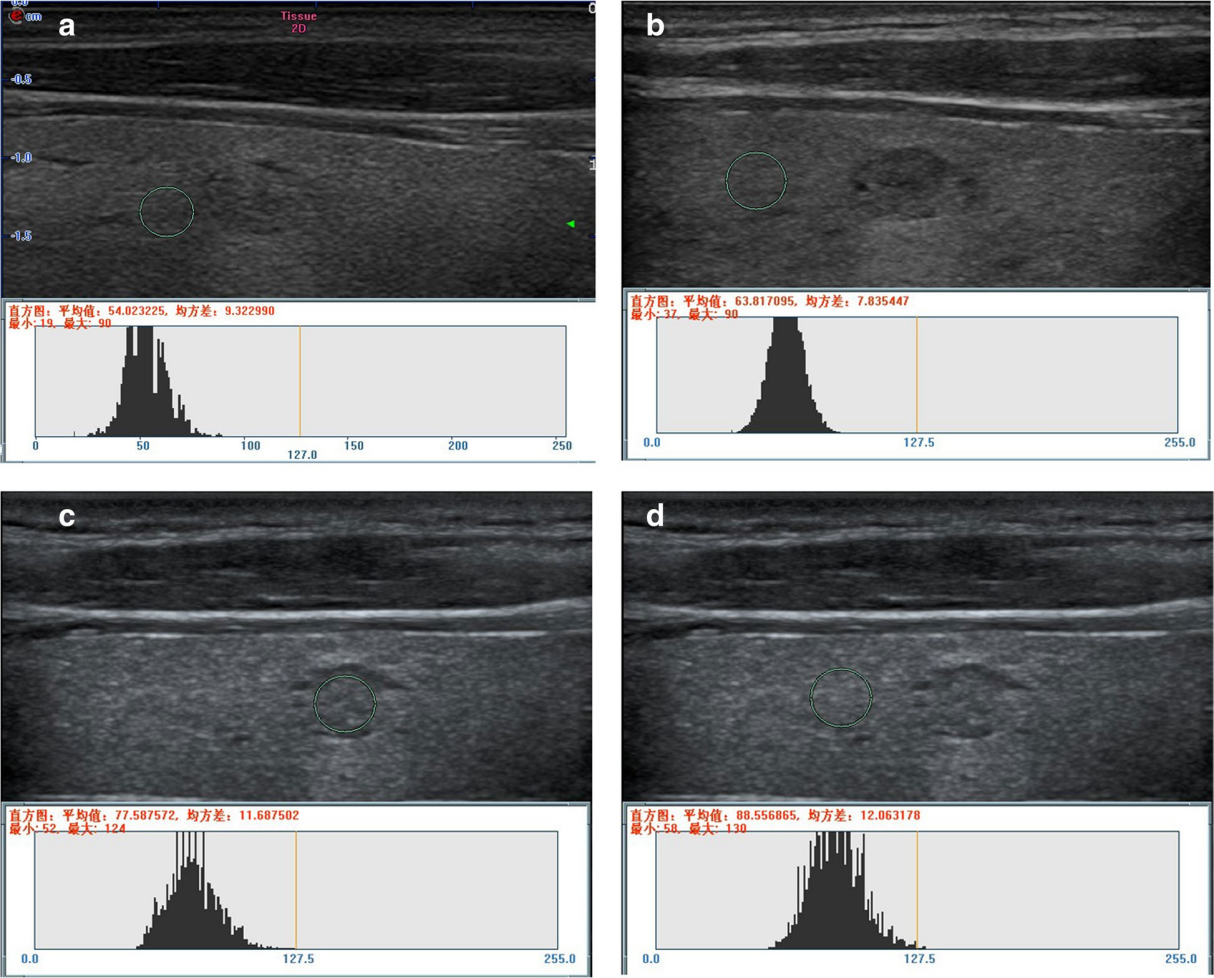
Measurement of the UGSR value for MNG on the left lobe of the thyroid. a and b (ultrasonic apparatus: Mindray Resona7): In the outpatient examination group, a longitudinal scan revealed grayscales of 54.667273 and 63.817095 for MNG and thyroid tissues at the same gain level, respectively. The UGSR value was 0.856624 (54.667273/63.817095). c and d (ultrasonic apparatus: Esaote Mylab 90): In the preoperative positioning group, a longitudinal scan shows grayscales of 77.587572 and 88.556865 for MNG and thyroid tissues at the same gain level, respectively. The UGSR value was 0.876133 (77.587572/88.556865), and the interval was 10 days. The difference of the UGSR was 0.019509 (0.876133–0.856624) between the two groups (the outpatient examination and preoperative positioning groups)
